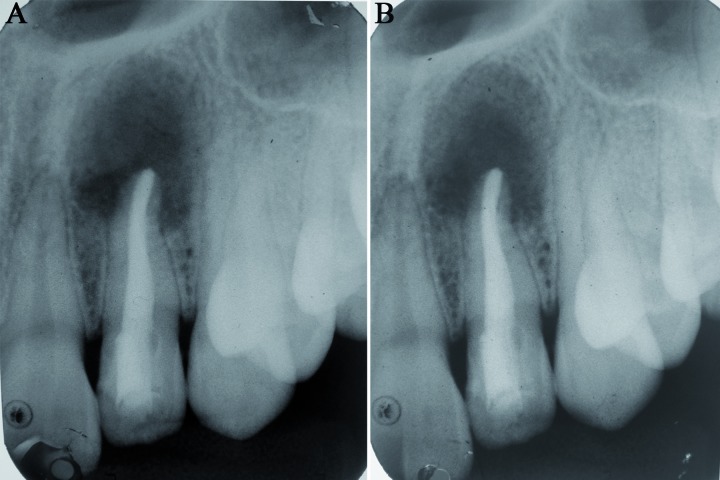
A) 6 week follow up radiograph; B) 12 week follow up radiograph showing bone formation from periphery to center.
